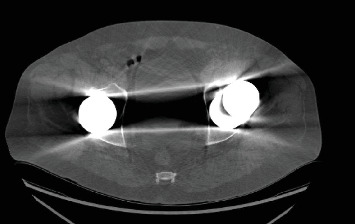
CT pelvis without contrast demonstrating increased acetabular component anteversion of the unstable left total hip arthroplasty.
